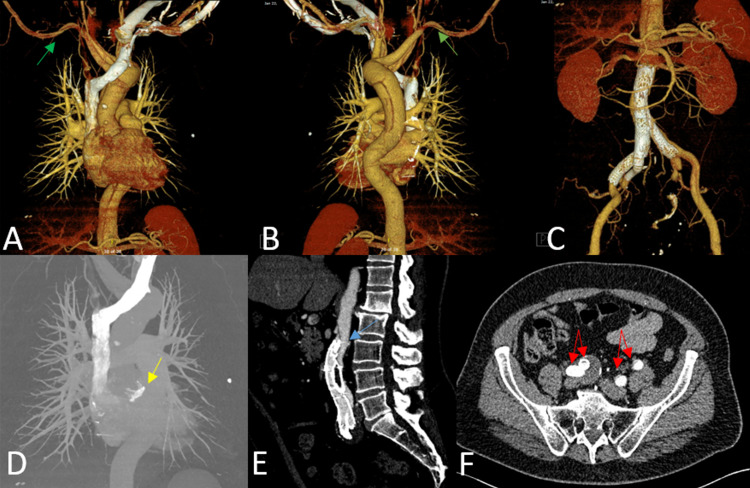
Six-Month Follow-Up Computed Tomography (CT) Imaging CT imaging of the thorax showing aberrant right subclavian artery (green arrow) branching off from the distal aortic arch with a retroesophageal course. (A) Anterior thoracic CT image with three-dimensional (3D) reconstruction. (B) Posterior thoracic CT image with 3D reconstruction. (C) 3D anterior abdominal CT imaging showing a bifurcated stent graft of the right common iliac artery excluding the right common iliac artery aneurysm. (D) Coronal thoracic CT image showing the aortic valve in place (yellow arrow). (E) Sagittal lumbar CT image showing that the proximal end of the endograft remained partially collapsed (blue arrow). (F) Axial abdominal CT showing no evidence of endoleak in the right iliac artery. Red arrows: bilateral internal and external iliac arteries opacified by contrast.
